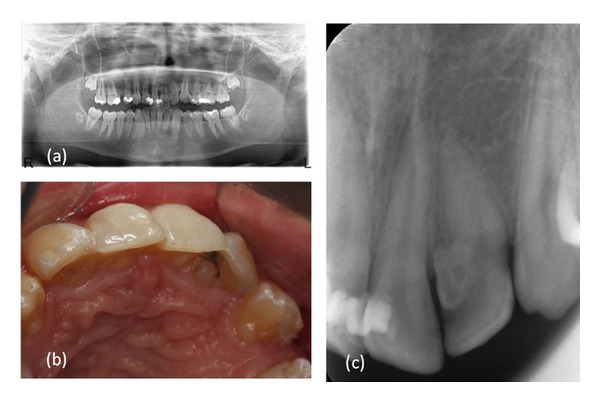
(a) Preoperative panoramic radiograph, (b) preoperative photograph: palatinal view, and (c) preoperative radiograph revealing the maxillary left lateral incisor with an unusual anatomy.
